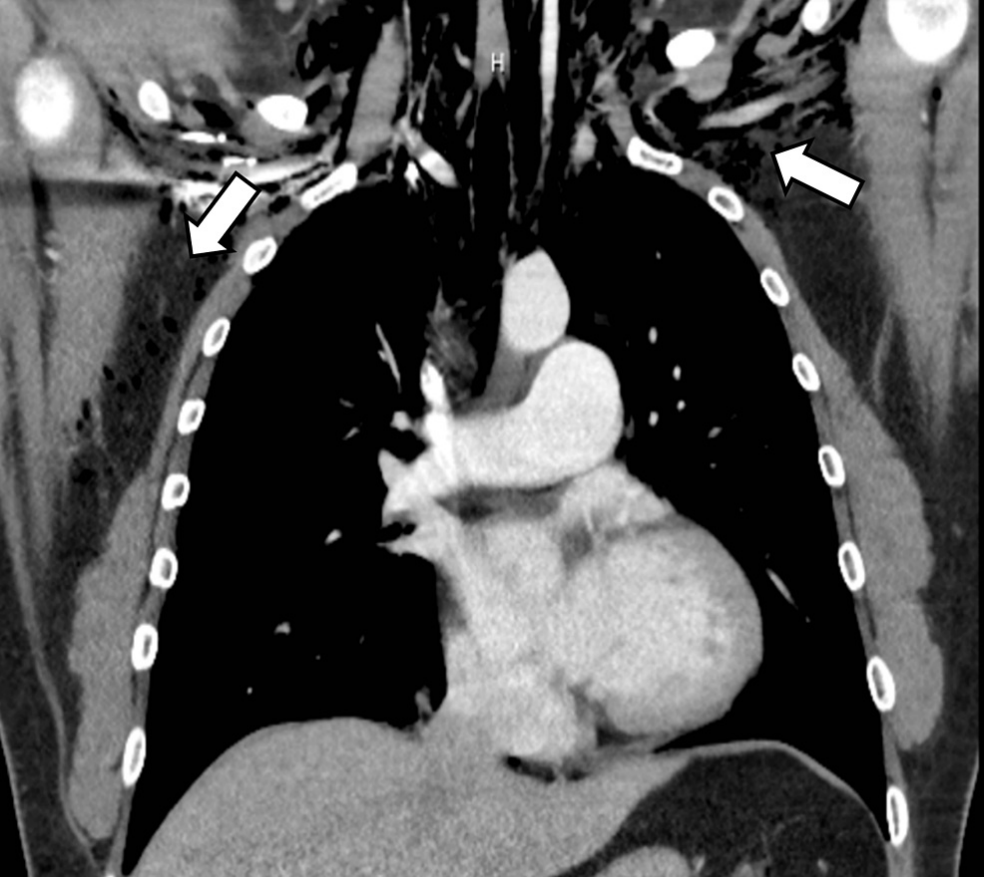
Chest CT with contrast showing extensive pneumomediastinum and subcutaneous air present in the chest wall and base of the neck. There are also bilateral apical pneumothoraces. The findings are consistent with the rupture of a small airway
